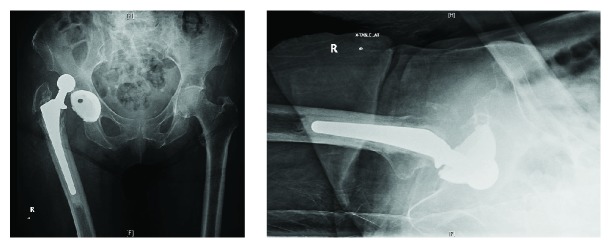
Anteroposterior (AP) pelvis and lateral right hip radiographs showing a posterosuperior dislocation of the right cemented femoral component with associated chronic greater trochanteric periprosthetic fracture and chronic left inferior pubic rami fracture.
